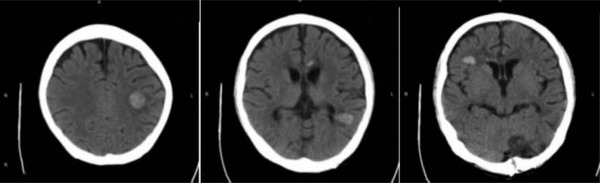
CT images of the patient's brain before transplantation. Multiple high‐density nodules and masses with peripheral edema in bilateral semiovale center, corpus callosum, bilateral paraventricular areas, right frontal lobe, and left cerebellar hemisphere
